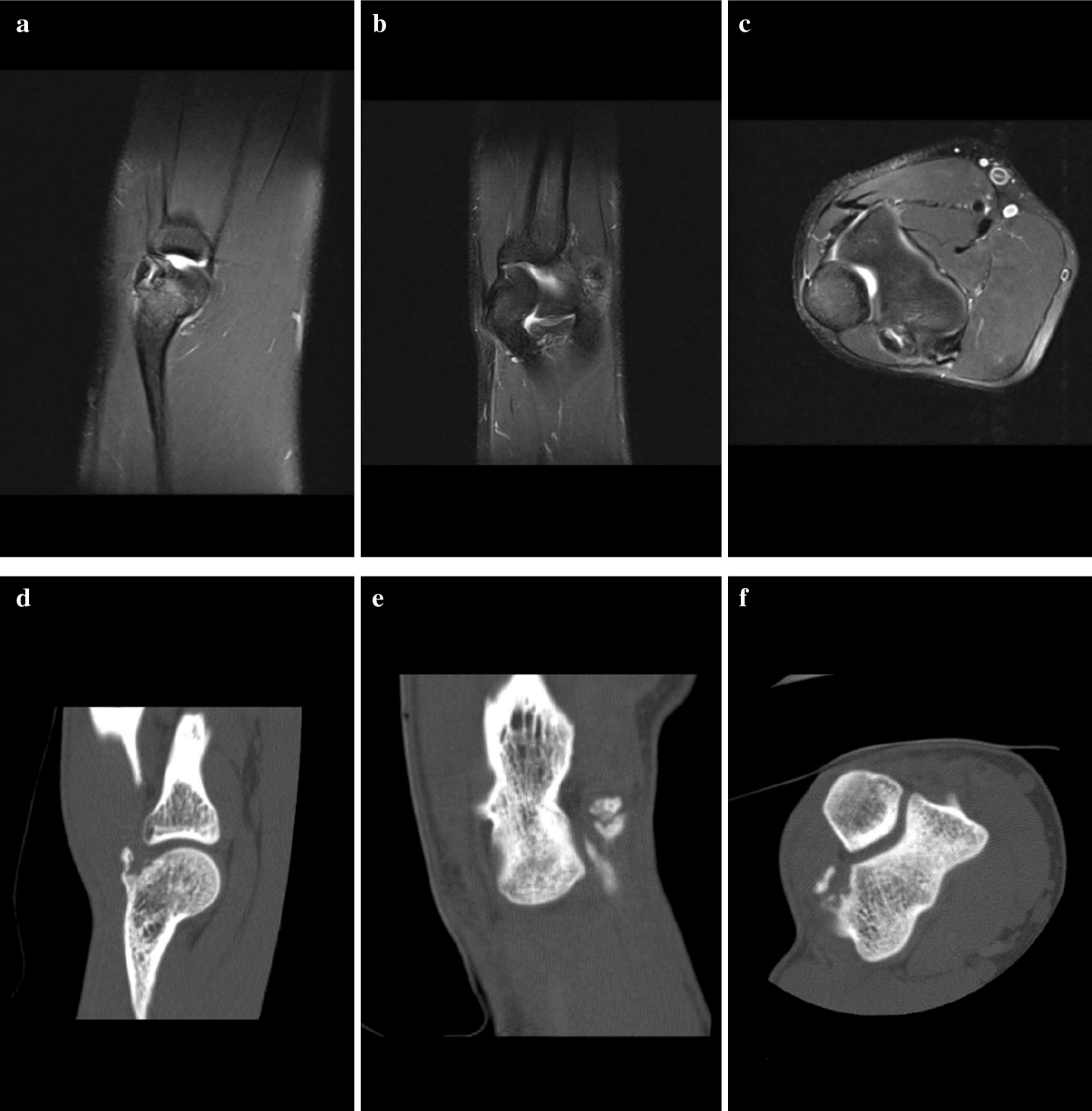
Comparison of MR images (a–c) and CT scans (d–f) of the right elbow of patient no. 11
